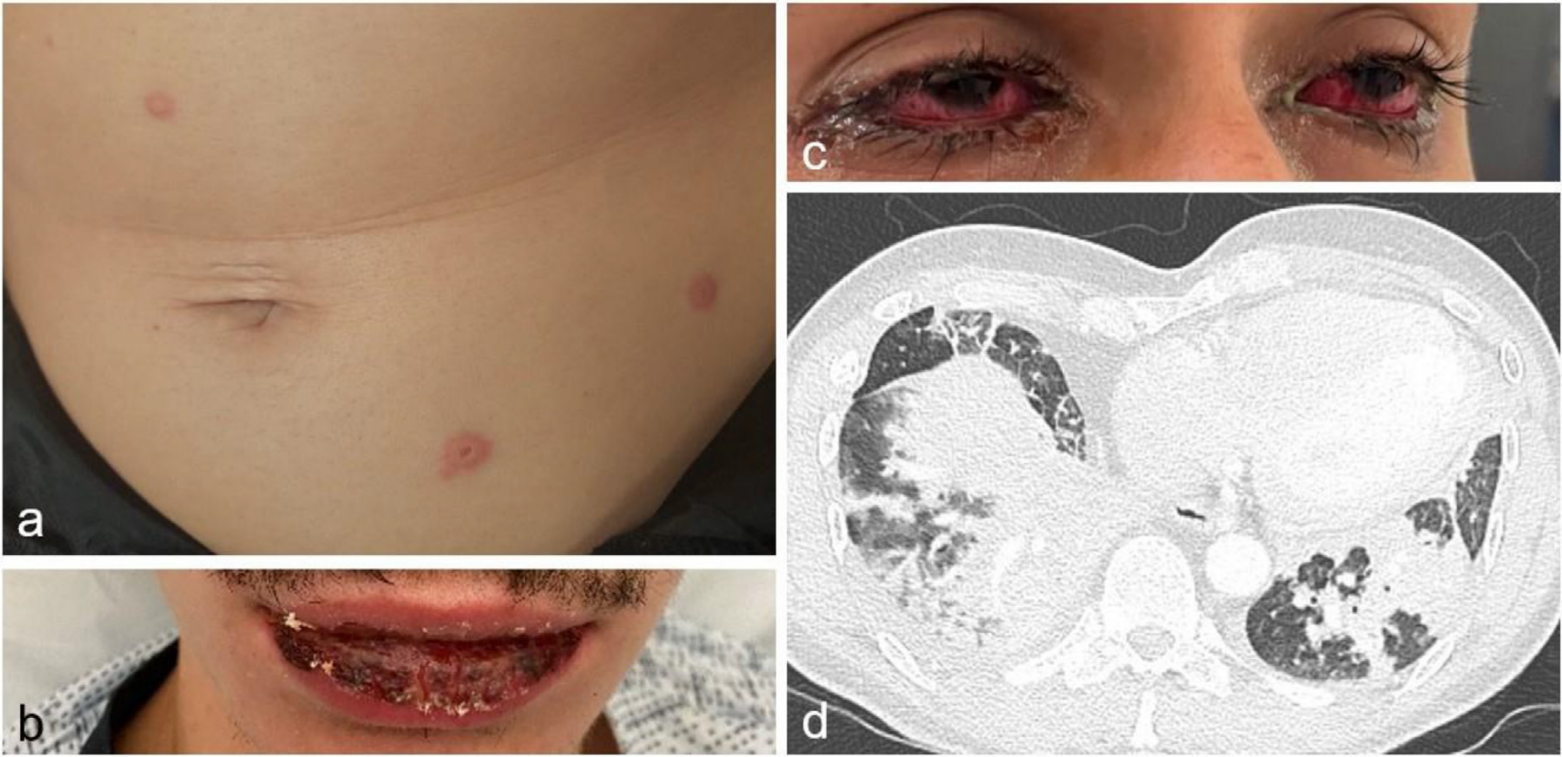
Extrapulmonary and pulmonary presentations of *Mycoplasma pneumoniae* infection. (a) Target-like lesions including a central bulla on the abdomen consistent with erythema multiforme in a 36-year-old female; (b) Mucositis in a 27-year-old male patient; (c) Bilateral conjunctivitis; (d) Bilateral *M. pneumoniae* pleuropneumonia with reactive mediastinal lymph nodes, in a 57-year-old male (axial computed tomographic-scan).
